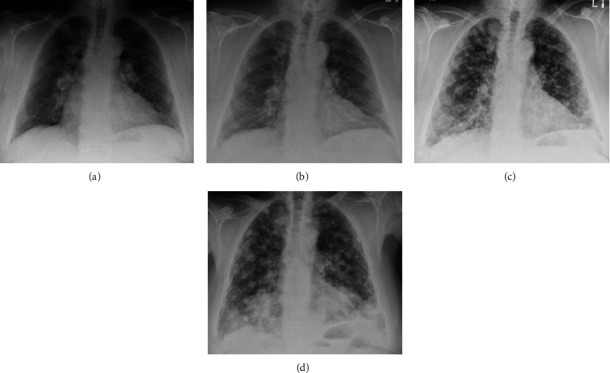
Chest radiographs of Patient 3 showing slow progression of metastatic nodules at two years (a), five years (b), seven years (c), and 10 years (d) of follow-up.
